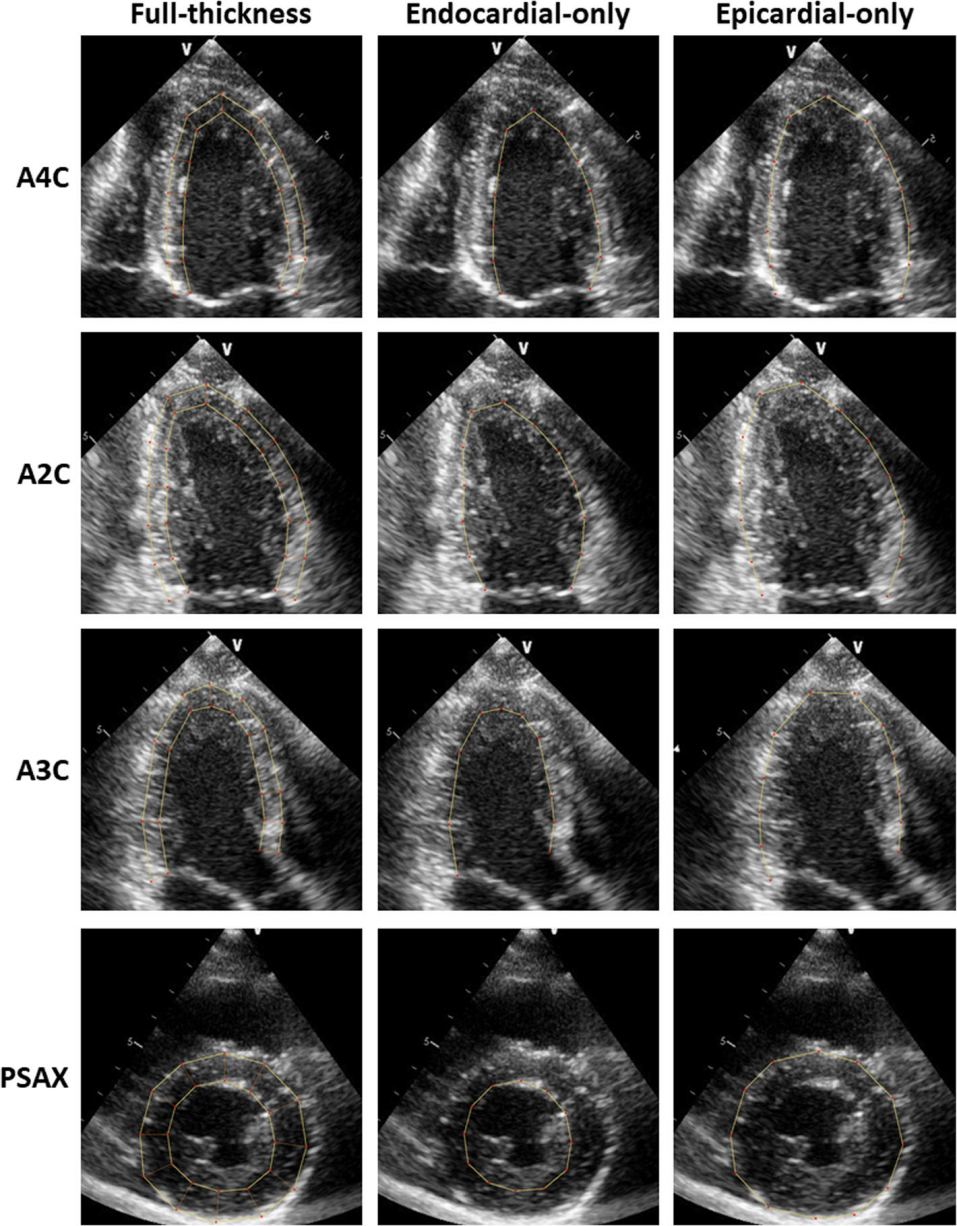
Layer-specific strain analysis of the left ventricle. Longitudinal strain measurements were obtained from the apical 4-chamber (A4C), 2-chamber (A2C), and 3-chamber (A3C) views. Circumferential and radial strain measurements were obtained from the parasternal short-axis (PSAX) view at the papillary muscle level. Full-thickness measurements were performed by tracing an inner border at the endocardium and an outer border at the epicardium. Endocardial-only strain measurements were performed using the same inner endocardial border as the full-thickness measurements. Epicardial-only measurements were performed by approximating the same outer epicardial border as the full-thickness measurements
